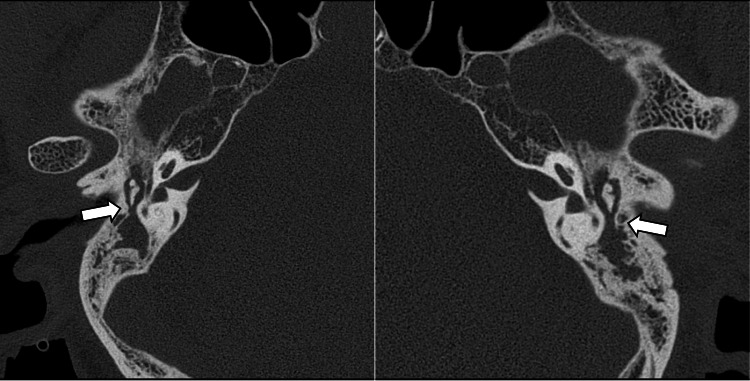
Axial computed tomography (CT) scan of the temporal bones. Bilateral mastoid hypopneumatization and soft tissue densities extending from the mastoid cavities into the tympanic cavities were observed (arrows).
